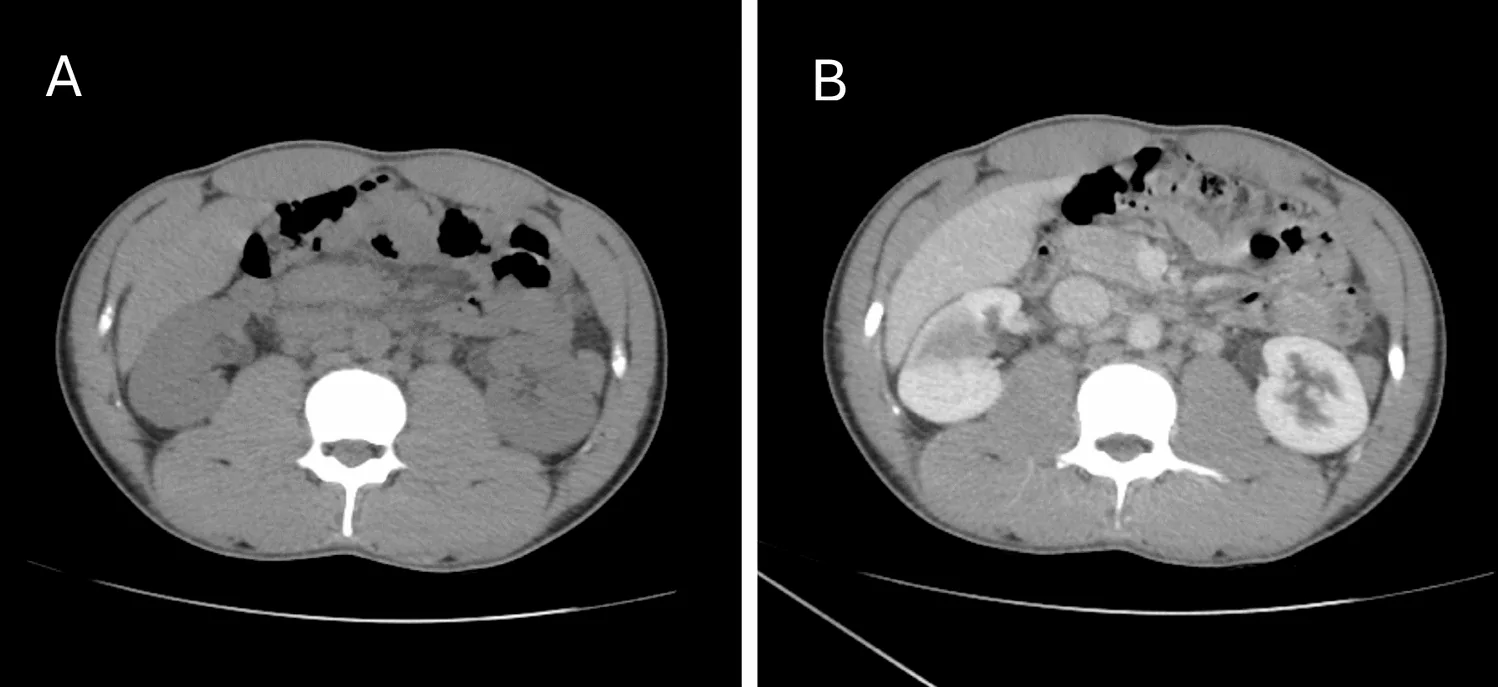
23-year-old Black male with sickle cell trait presenting with haematuria. After a normal ultrasound, he re-presented one month later with haemoptysis and chest pain and was diagnosed with a pulmonary embolus. Enlarged lymph nodes were noted above and below the diaphragm. An 18F-FDG PET/CT did not detect the primary tumour. A) Non-contrast-enhanced and B) contrast-enhanced CT scans show a 3.2 cm hypoenhancing right interpolar renal mass protruding into the collecting system, with retroperitoneal lymphadenopathy
